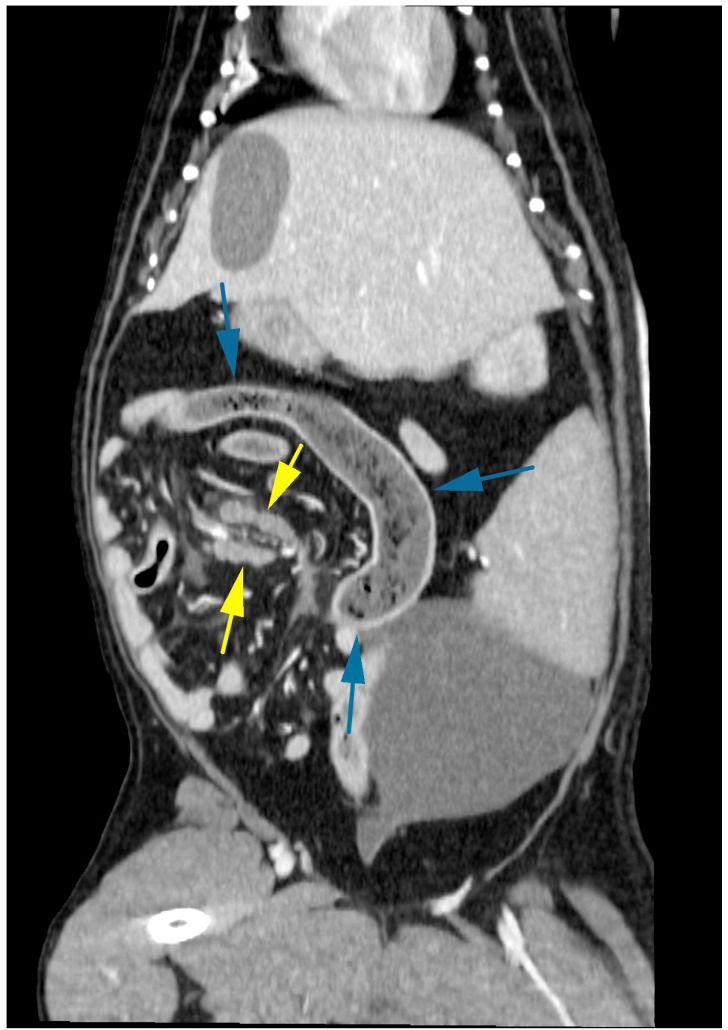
Abdominal CT in the transverse plane revealed a moderately dilated segment of the jejunum with mixed heterogeneous gas and soft tissue opacity (blue arrows), with moderate enlargement of the adjacent lymph nodes (yellow arrows).
